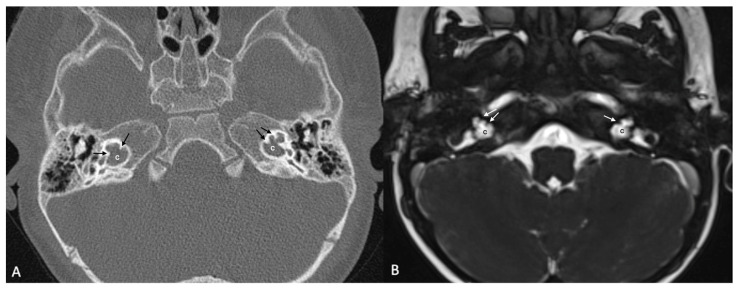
(A) CT and (B) MRI images of a case of bilateral IP-3 (incomplete partition type-3). Note that despite the relative preservation of the outer contour of both cochleae (c) and the presence of interscalar septae (black and white arrows), the inner structure is featureless and the modiolus is absent.
